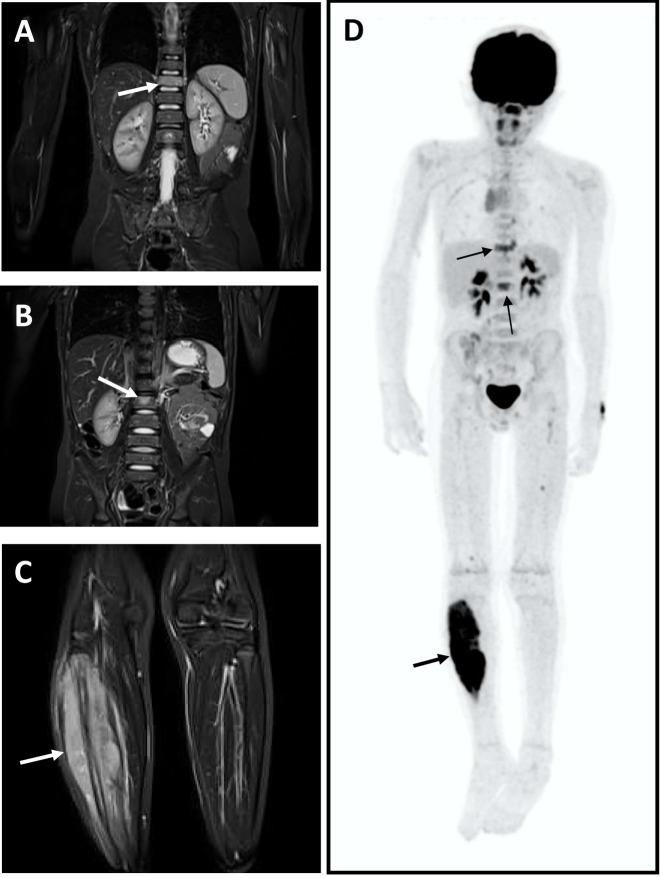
Nine-year-old boy with primary Ewing sarcoma of the right fibula and spinal bone metastases (A–C). Coronal STIR MR images from a whole-body MRI study show the primary tumour (arrow—C) and metastases in the spine (arrows—A,B). Coronal PET study demonstrates intense FDG uptake in the right fibula (D) (thick arrow) and also in the spinal metastases (thin arrows).
